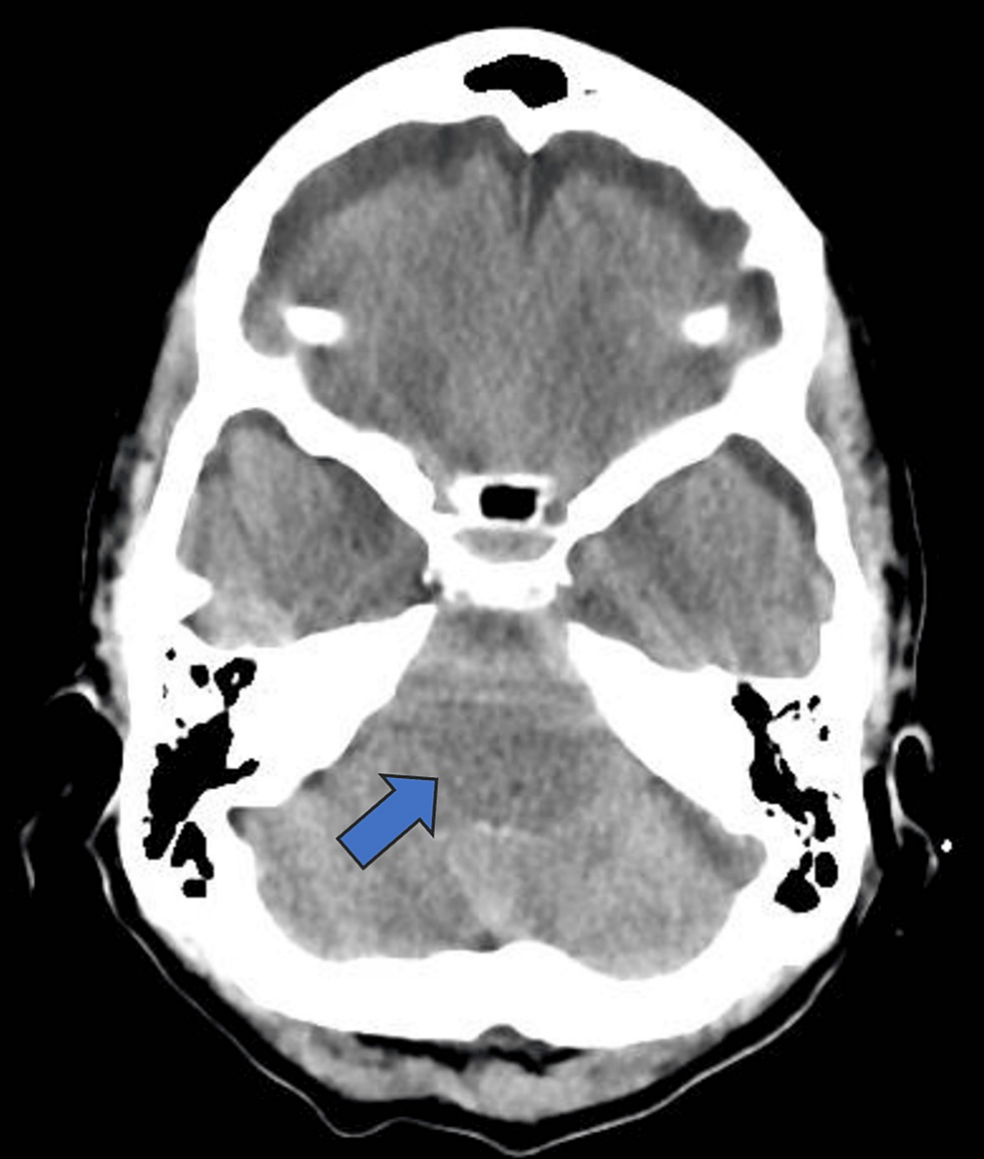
CT scan head showing effacement of basilar cisterns (arrow)
